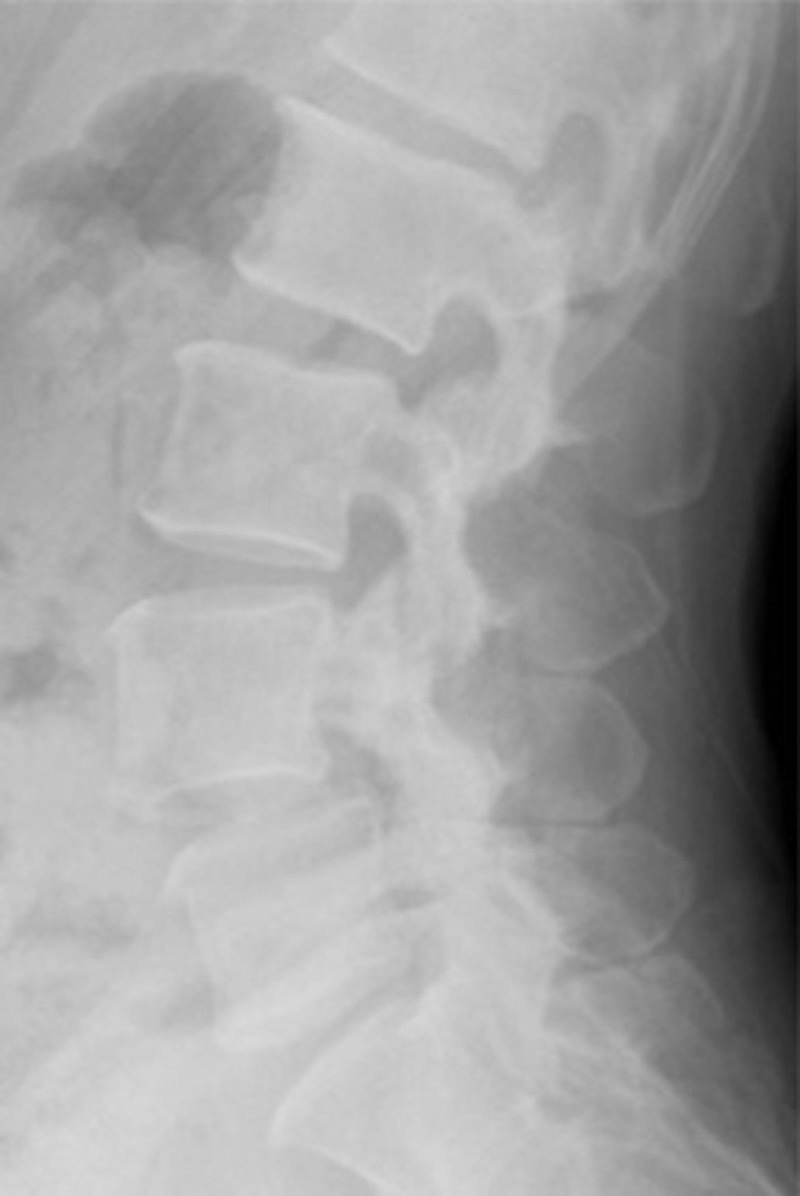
X-ray of a Strong Candidate Lateral x-ray shows good bone quality, which aids interbody fusion.
